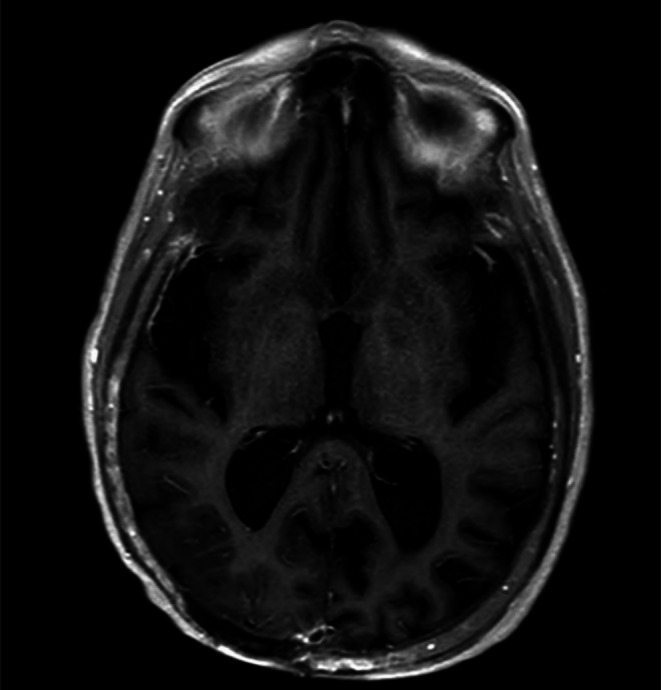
Contrasted MRI Brain showing resolution of cortical gyriform enhancement on repeat imaging.
